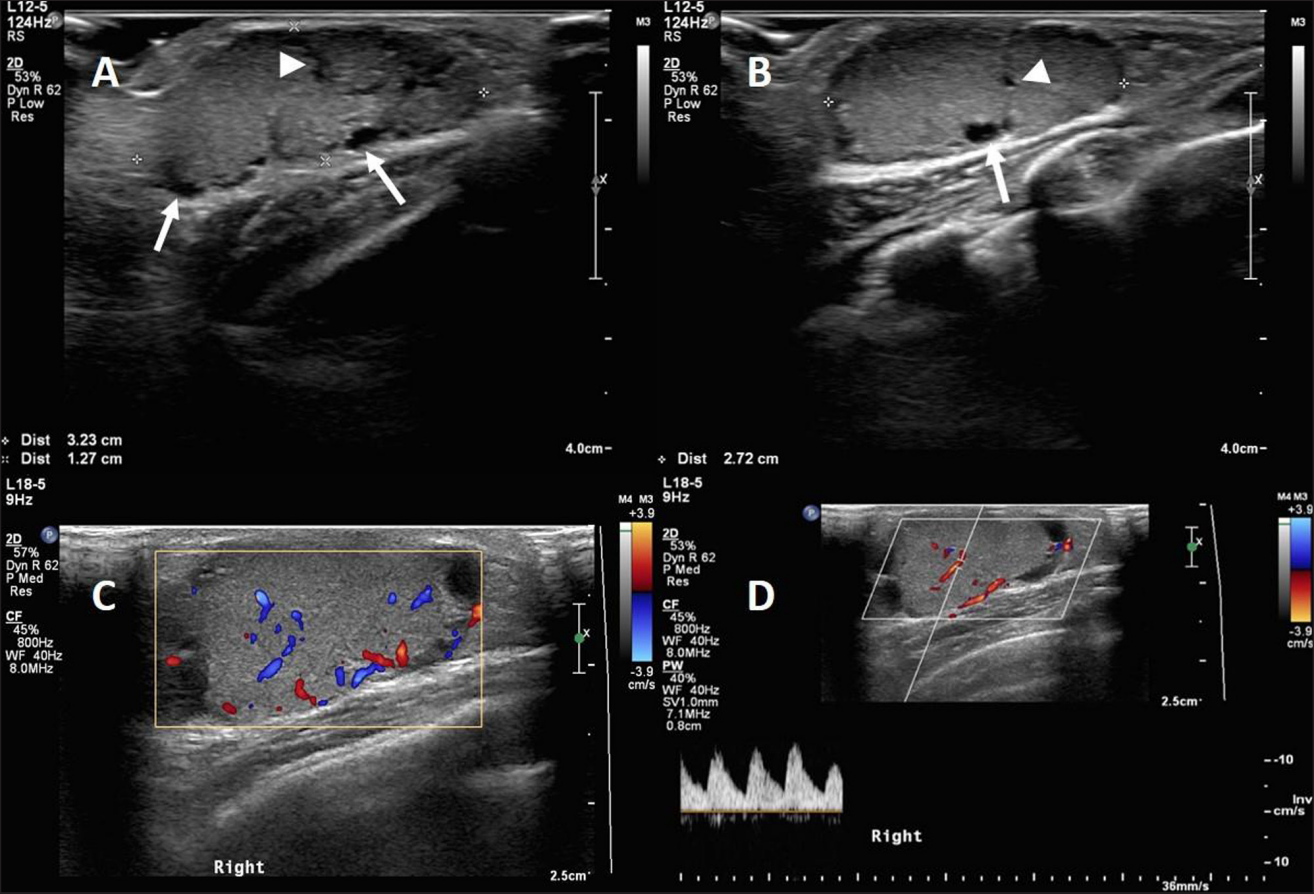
Ultrasound examination (A–D) demonstrates a well-defined, heterogenous lesion (3,2 cm LL × 1,3 cm AP × 2,7 cm CC) with sharp boundaries. Note the peripheral cysts (arrows) and hypoechoic clefts (arrowheads) (A–B). The mass is vascularized (C–D), predominantly peripheral, with detectable arterial flow.
